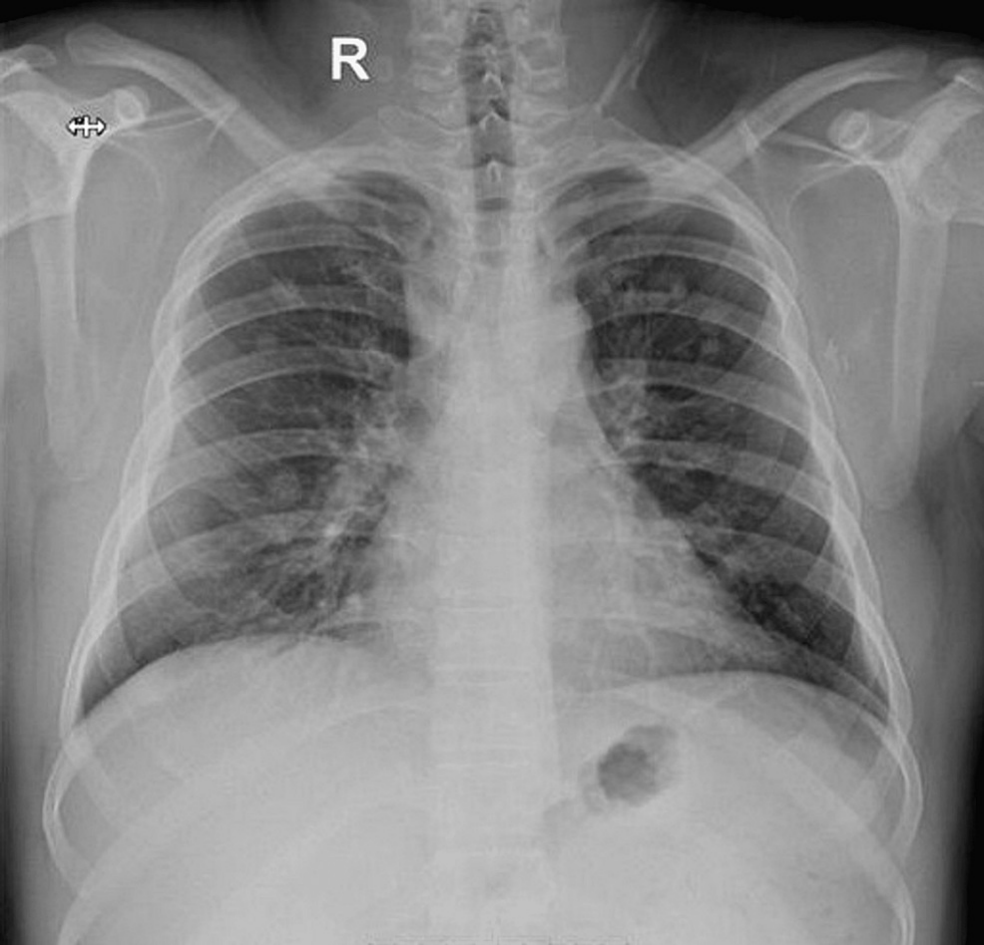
Chest X-ray done as a part of follow-up imaging. Interval development of multiple bilateral nodular lesions in the lungs.
